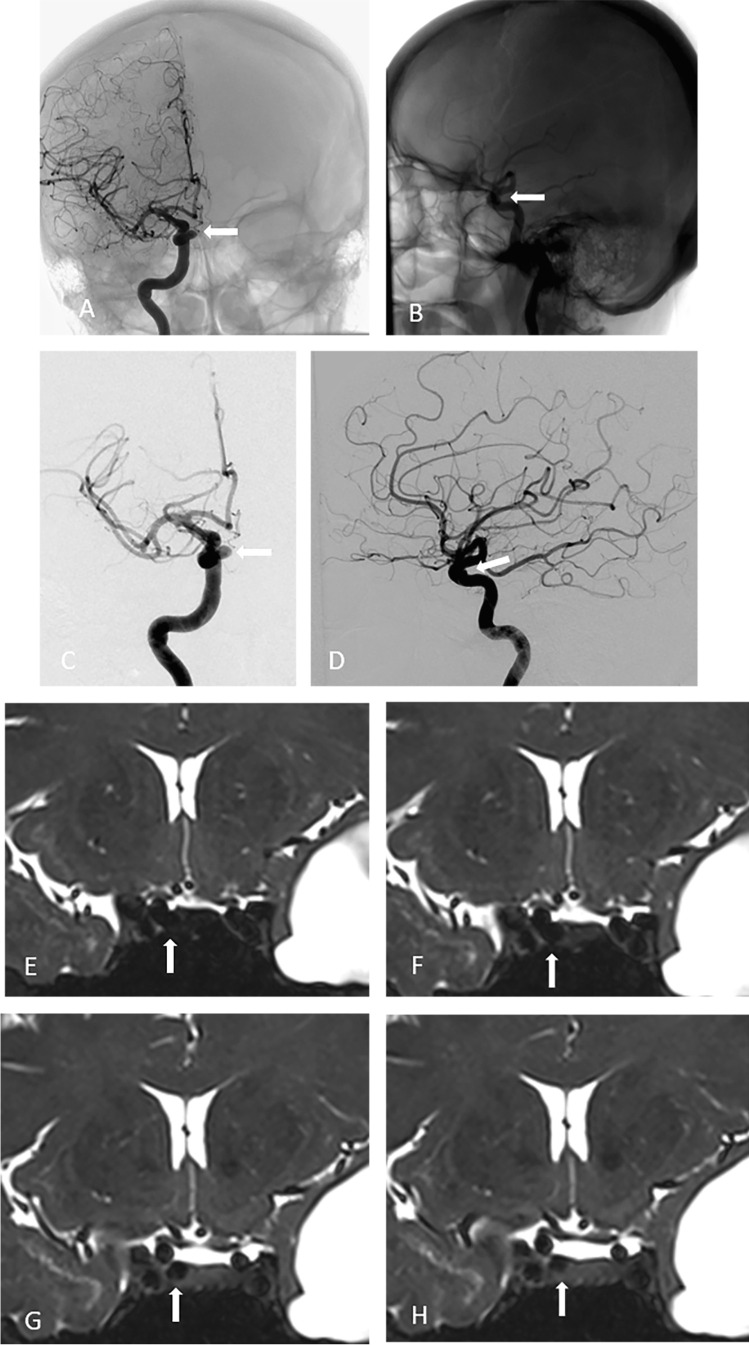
Cerebral digital subtraction angiography (DSA) of intracavernous aneurysm #19 (see Table 1) showing anteroposterior (panels A and C) and profile (panels B and D) views. Note the medial projection of the aneurysm (white arrows). Coronal T2-weighted magnetic resonance imaging (MRI) scans with a 3D fast spin-echo sequence showing anterior to posterior views of the anatomoradiological markers of the paraclinoid region and intracavernous aneurysm #19 (panels E–H). Note the hyper-intense signal from the cerebrospinal fluid around the distal dural ring and no contact between CSF and the aneurysm (arrow heads)
